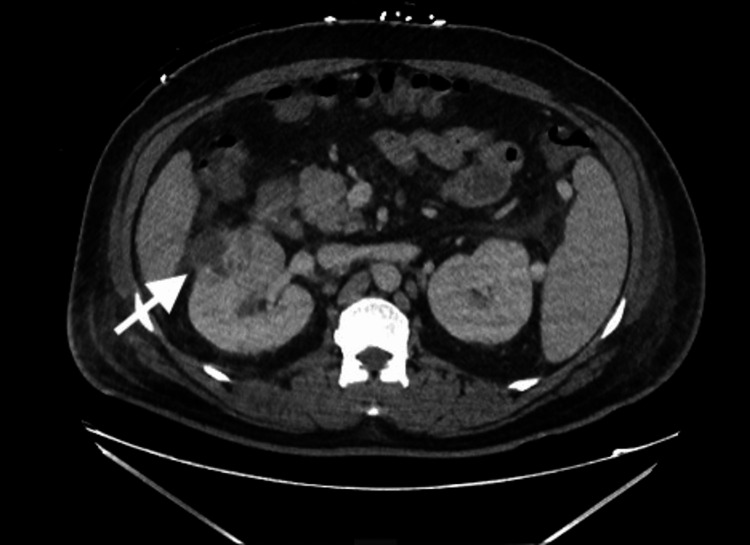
CT abdomen and pelvis showing a 4 cm heterogeneously enhancing right renal mass suspicious for renal cell carcinoma.
